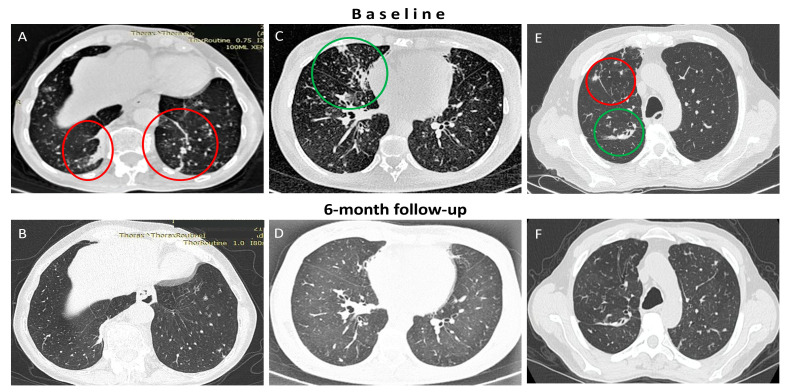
Radiological improvement of patients on treatment with ALIS for NTM-LD. Six months after ALIS initiation, all patients with MAC-associated LD (Panels (A–D)) and some patients with *M. abscessus-LD* (Panels (E,F) presented an improvement in CT findings such as nodules (red circles) and consolidations (green circles). (*ALIS: Amikacin liposomal inhalation suspension, MAC: M. avium complex, NTM: non-tuberculous mycobacteria, LD: lung disease*).
